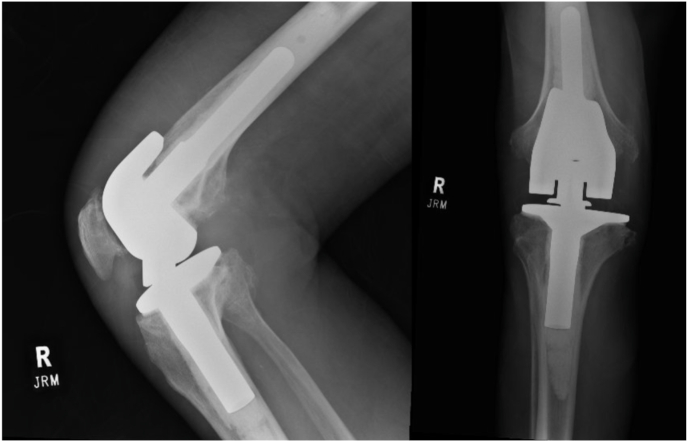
Preoperative Anteroposterior and Lateral radiographic views of knee prosthesis.
